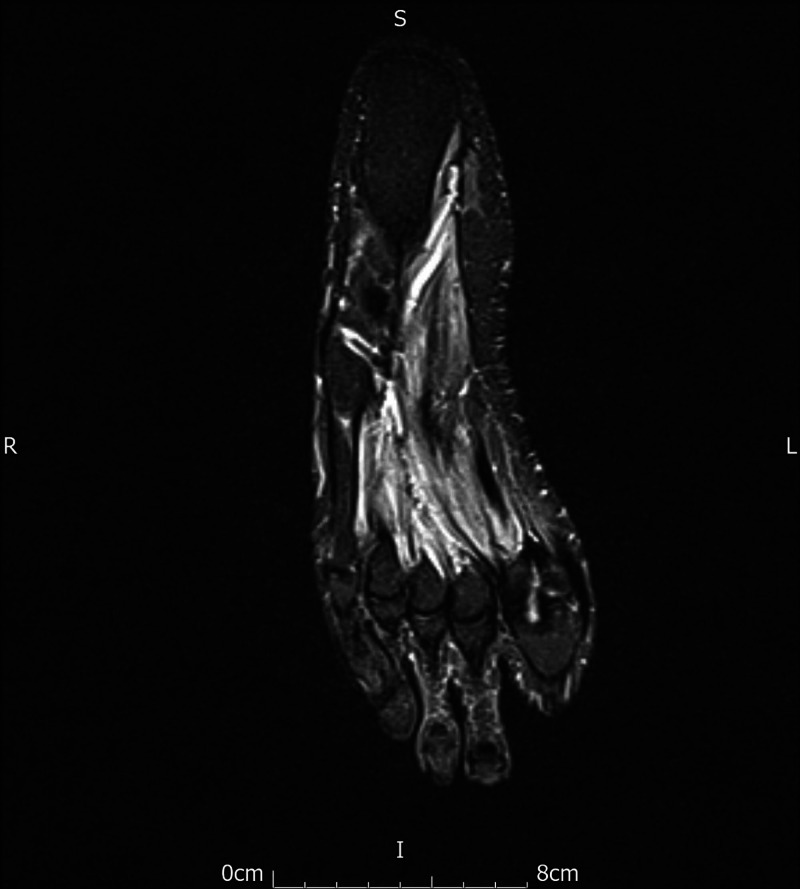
MRI right foot showing resolution of stress-related marrow oedema.
